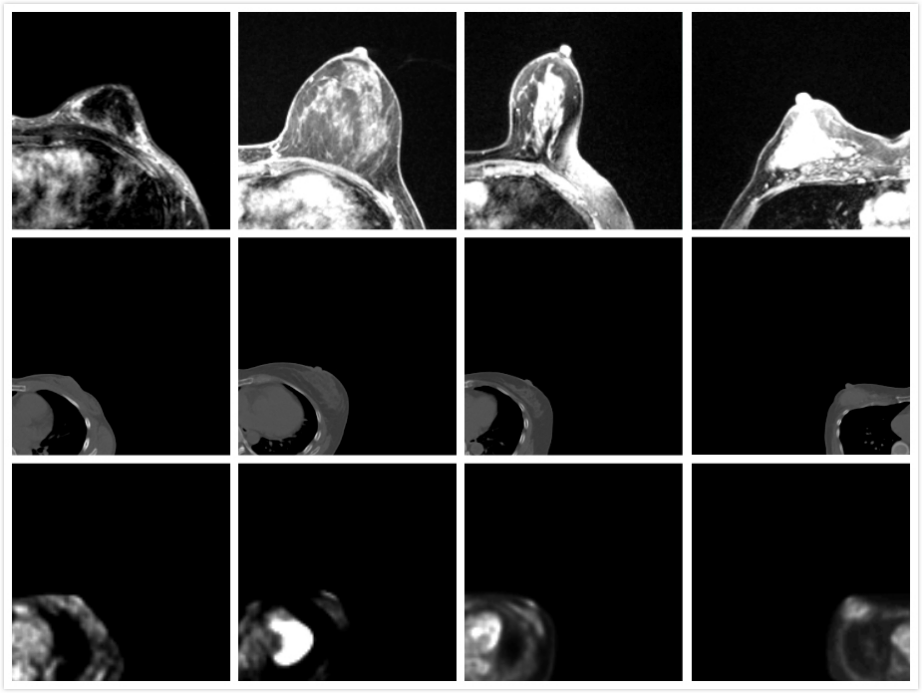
Appearance of MRI (first row), CT (second row), and PET images (third row). The direction of MRI is adjusted.
